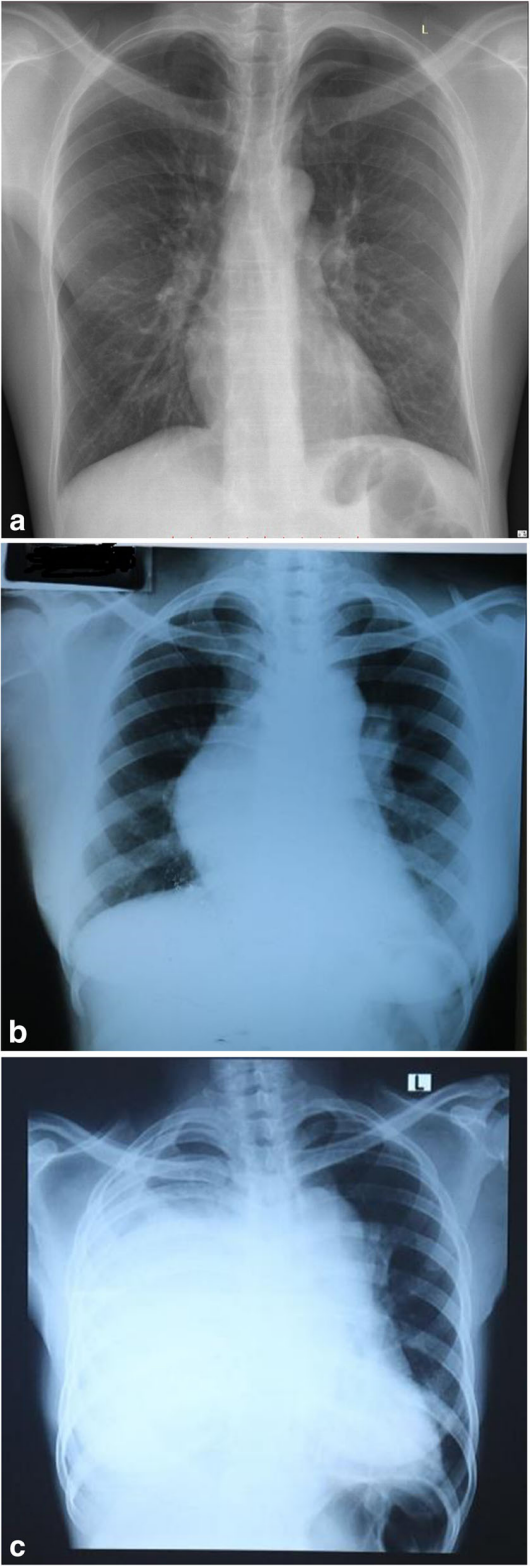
a Chest X-ray (posteroanterior view) taken 35 weeks prior, showing normal findings. b Chest X-ray (posteroanterior view) taken 21 weeks prior, showing right-sided mediastinal widening. c Chest X-ray (posteroanterior view) taken during the index admission, displaying right-sided homogenous opacification and left-sided tracheal deviation
